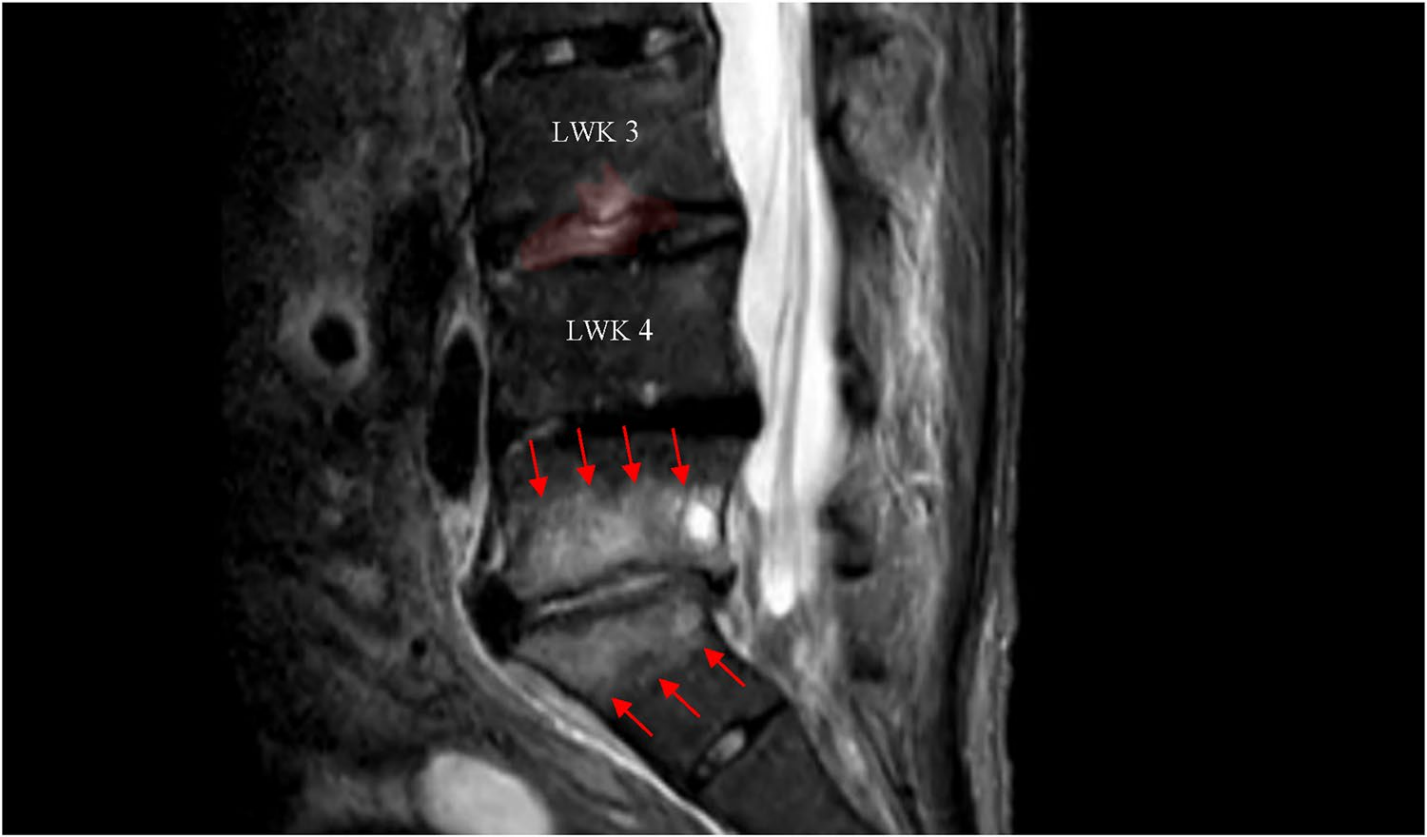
Characteristic MRI of VO in L3/4 and L5/S1. MRI of the lumbar spine of a patient presenting to the emergency department with fever, severe back pain, and elevated inflammatory markers (C-reactive protein 74 mg/L, leukocytes 12.000/µL). On STIR sequences, there was signal enhancement in the intervertebral disc and the superior and inferior endplates of the L5/S1 motion segment, accompanied by corresponding contrast enhancement (highlighted with arrows). Additionally, there was mild contrast uptake in the adjacent paravertebral soft tissues. In light of the clinical presentation, VO was strongly suspected in the L5/S1 segment. Minor changes were also observed in the L3/L4 segment (highlighted in red colour), where early inflammatory changes were also possible. *Enterococcus faecalis* was detected in blood cultures and in the intraoperative samples of the L3/4 and L5/S1 discs and verified the diagnosis of VO
